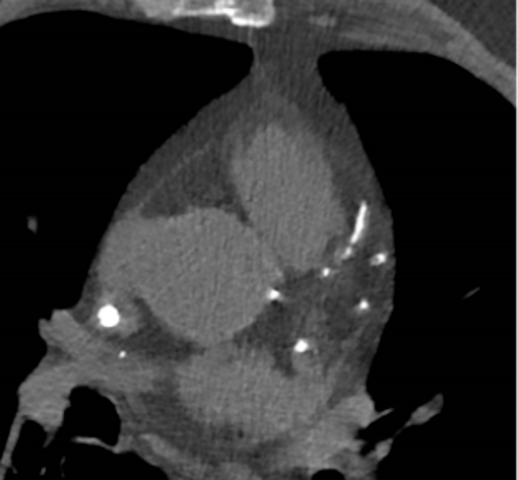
Coronary artery calcifications observed in computed tomography
